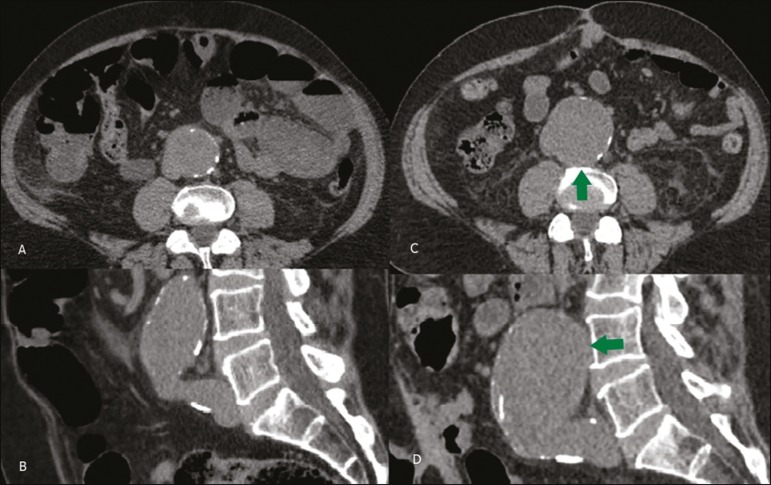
Unenhanced CT scans of a 62-year-old male patient in whom an AAA
